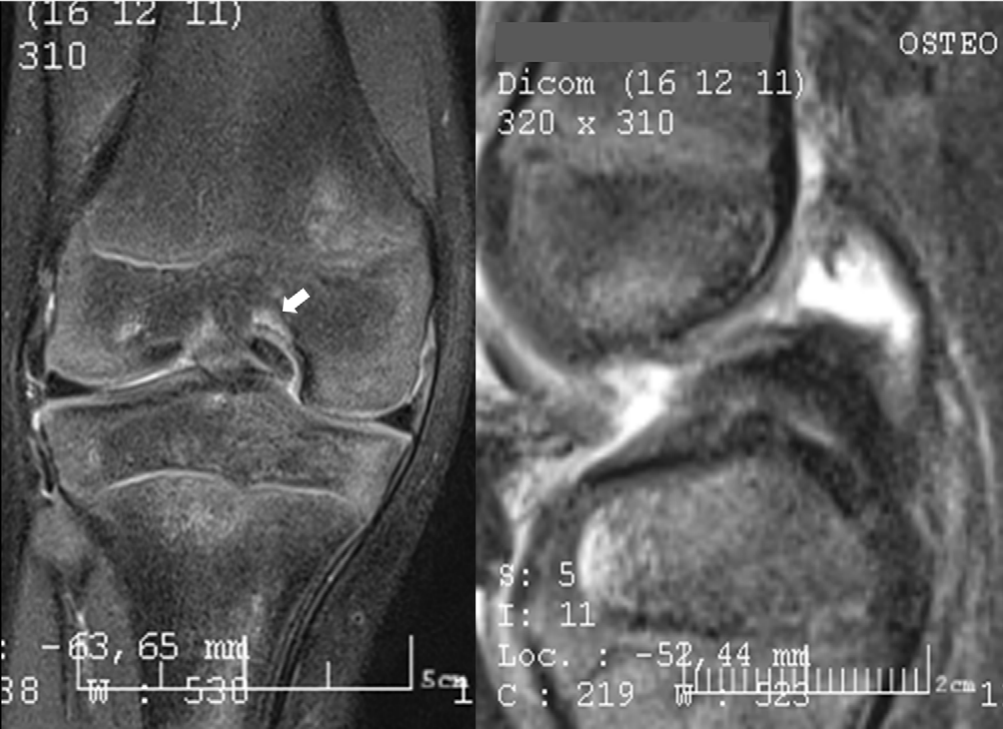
Case 1. MRI aspect after second assessment reveals cartilaginous nodule in the intercondylar notch with femoral oedema in coronal plane (left) and abnormal thickening of the proximal part of the PCL (right).Figure 3 F3:
